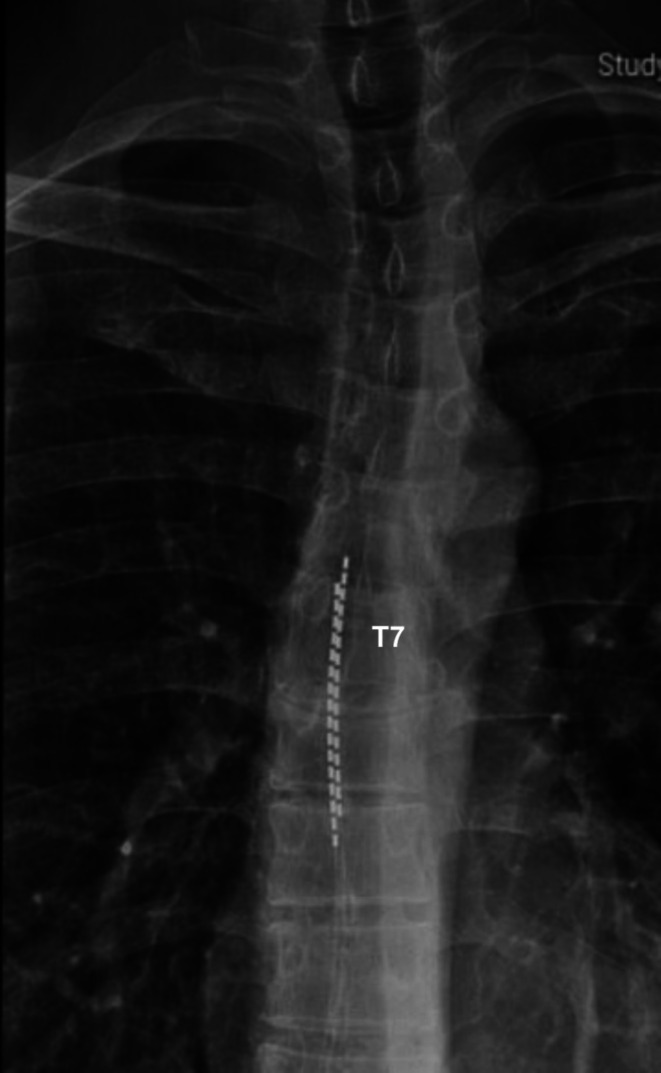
Anteroposterior thoracic plain film revealing caudal migration of the SCS lead, ending at the superior endplate of T7, and demonstrating anatomical rotational scoliosis.
